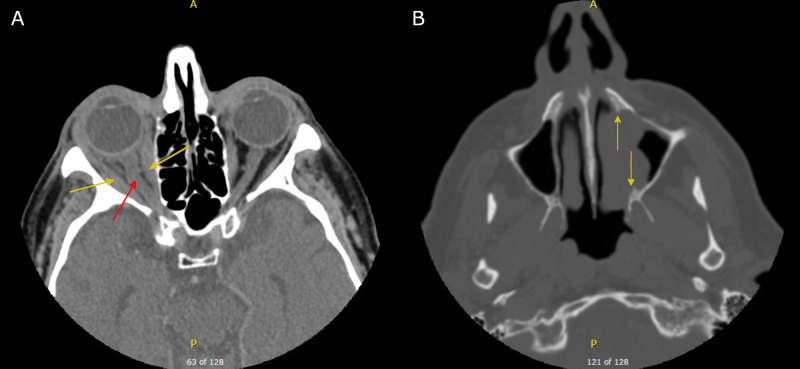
Non-contrast axial CT of the head and orbits. A) Soft tissue window showing diffuse enlargement of right extraocular muscles (yellow arrows) and tortuous right optic nerve (red arrow). B) Bone window showing a left-sided nasal tumor extending into the left maxillary sinus, with yellow arrows marking the extent of bone erosion. The coronal and sagittal reformats had incomplete visualization of the tumor vertically and are not shown.
